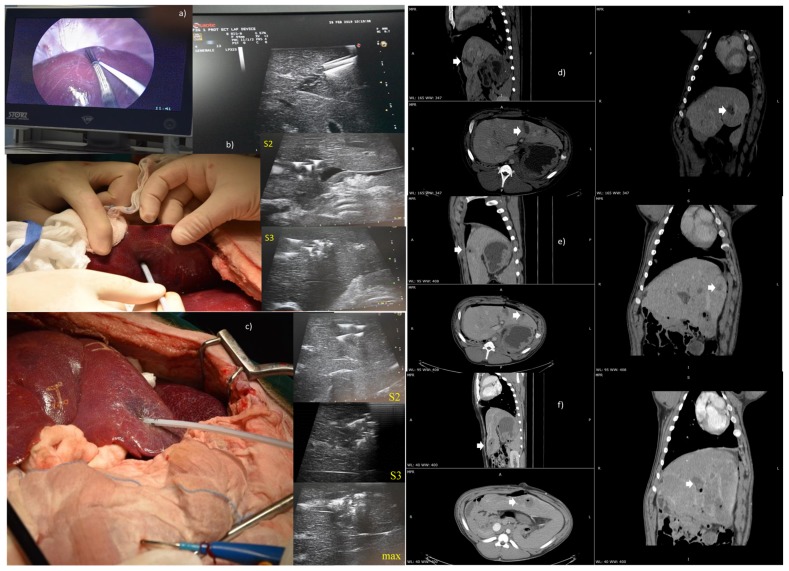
(a) Treatment performed by the laparoscopic access and ultrasound images used as a guide for the insertion of a 5-needle expandable electrode with zero divergence; (b) treatment in open surgery with a 5-needle expandable electrode prototype and ultrasound vision of the area treated in the two deployments (S2–S3); (c) treatment in open surgery using a 4-needle expandable electrode prototype (without central needle) and ultrasound vision of the area treated in the three deployments; (d) CT Multiplanar reconstructions of the ablated area with the 5-needles expandable electrode with zero divergence; (e) CT Multiplanar reconstructions of the ablated area with the 5-needles expandable electrode prototype (20° of divergence) and (f) with the 4-needles expandable electrode prototype (10° of divergence).
